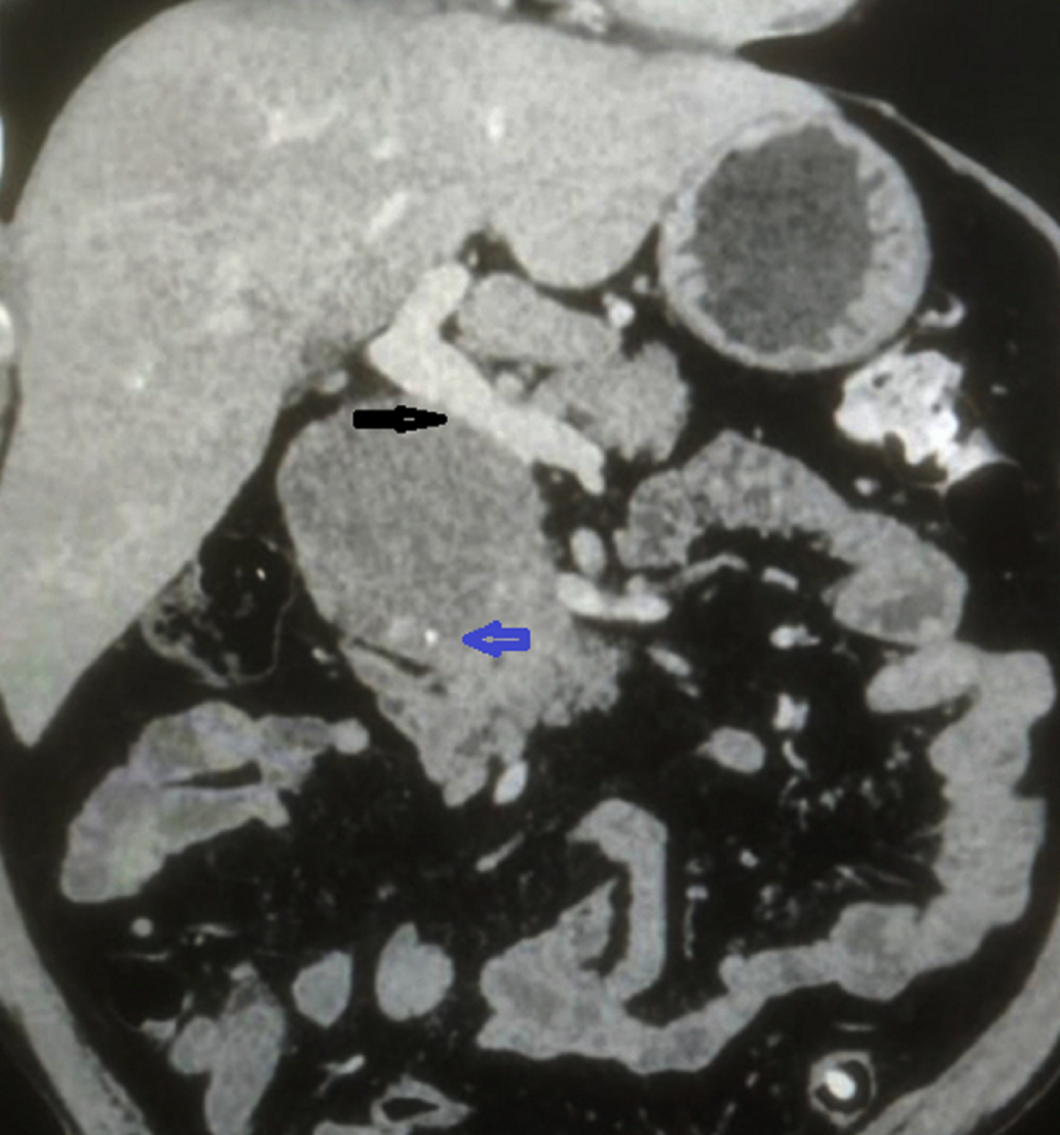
CECT scan of the abdomen, coronal view, showing the lesion closely abutting the portal vein with discontinuity of capsule (marked by black arrow), and peripheral calcification (marked with blue arrow) CECT: contrast-enhanced computed tomography
